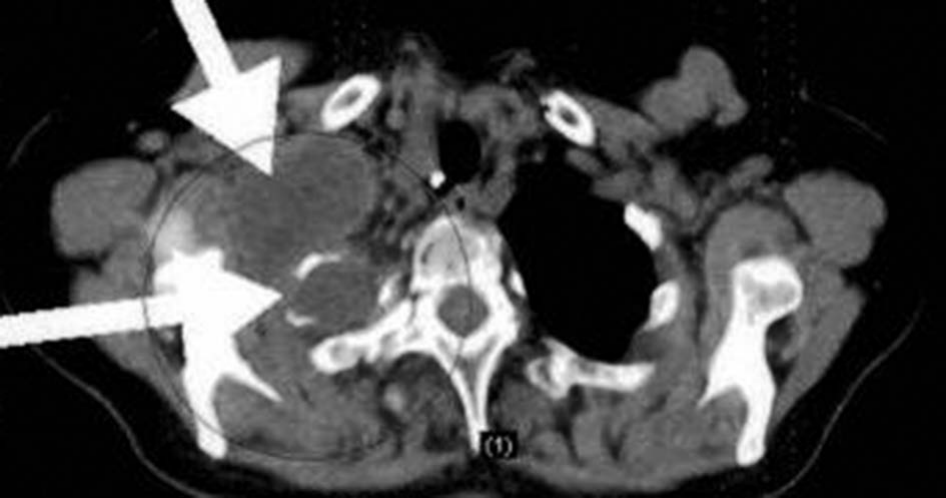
Preoperative PET/CT Image
